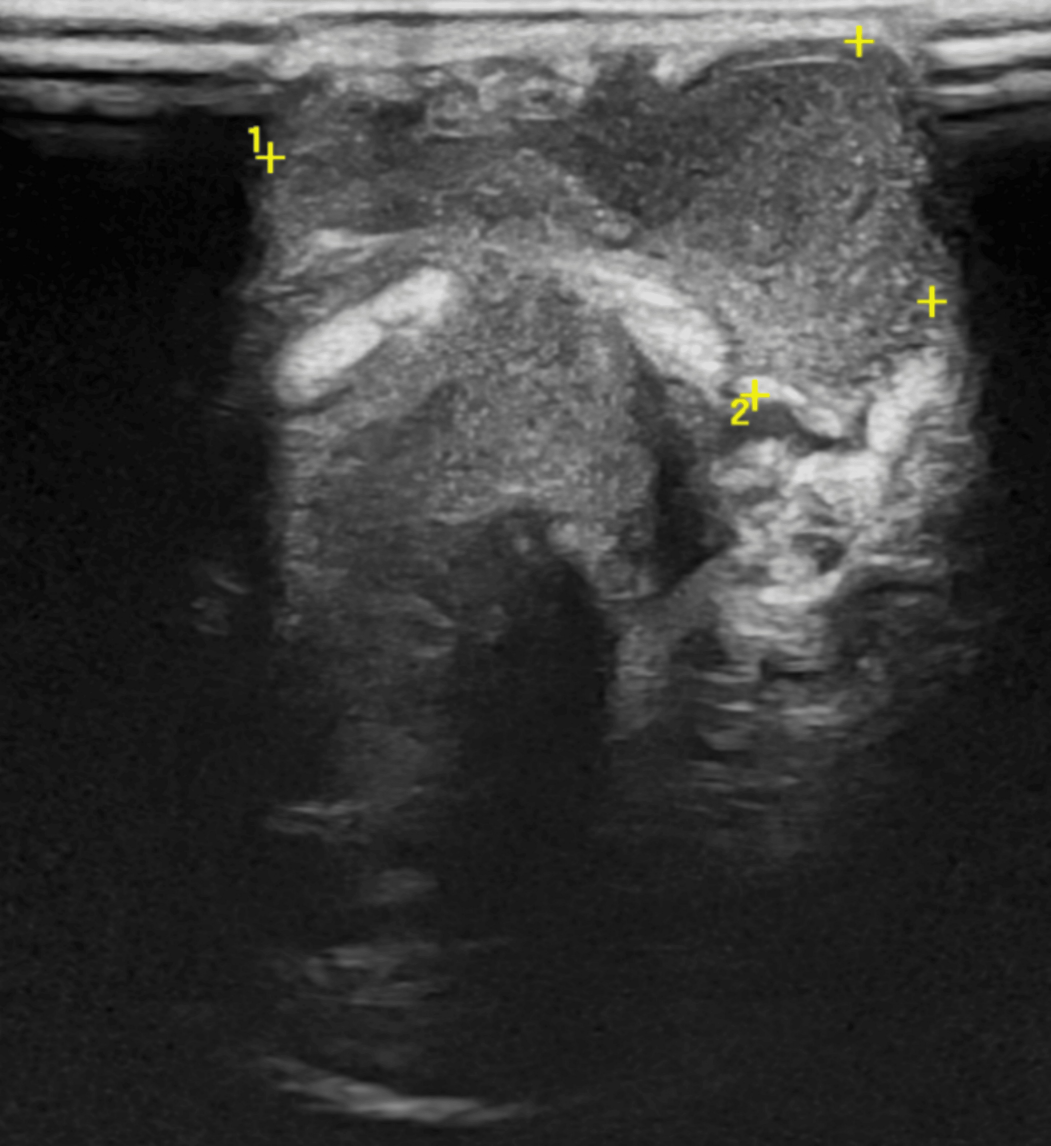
Right wrist ultrasound showing soft tissue abscess Ultrasound image demonstrating a heterogeneous collection located within the subcutaneous tissue and deep planes. Yellow calipers and numbers 1 and 2 demarcate the boundaries of the lesion (18 x 16 x 9 millimeters).
